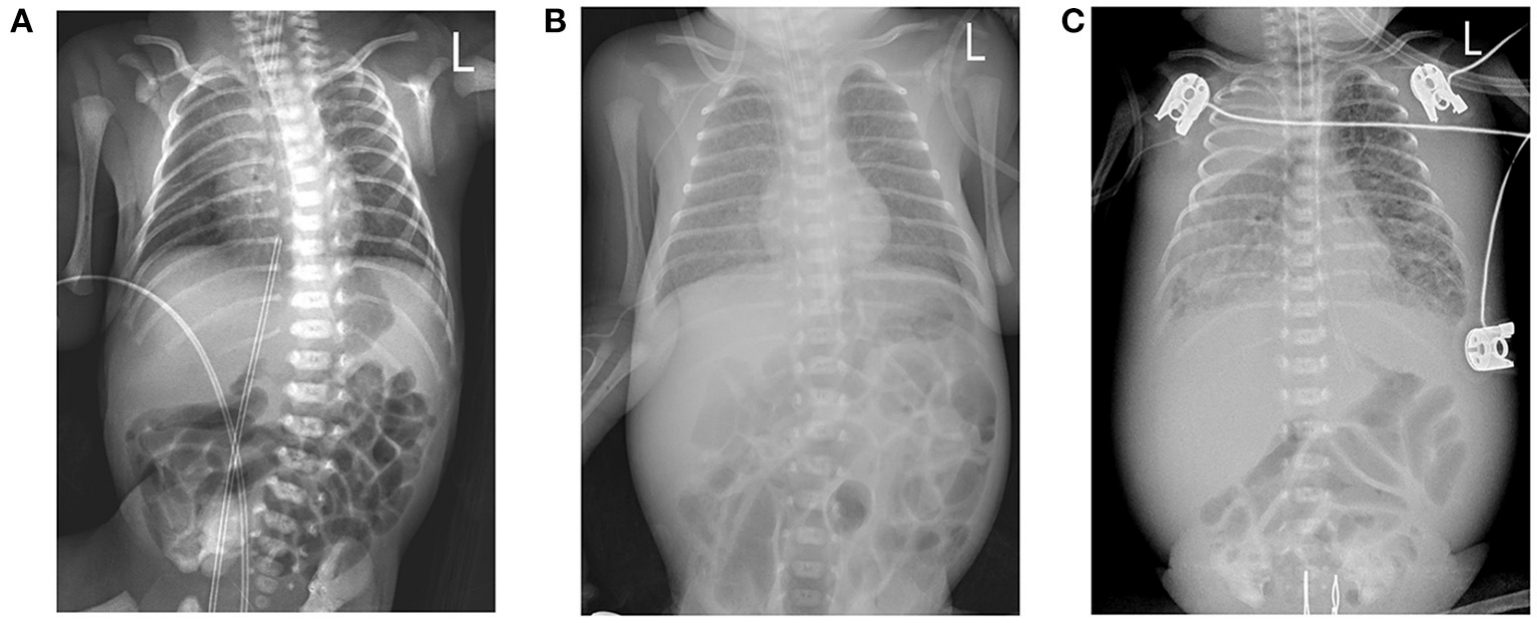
(A) Chest X-ray image shows a bilateral thickening of lung markings, with patchy and blurred shadows in the lower lung fields. (B) Chest X-ray image depicts thickening and blurring of bilateral lung markings, with small patches of fuzzy shadows in the lung fields. (C) Chest X-ray image shows that the texture of both lungs was thickened, increased, and blurred. Large dense shadows of uniform density were observed in the right upper lung field, and small patchy blurred shadows were found in the remaining lung fields. There was a narrow band of increased density in the field of the right lower lung that contained a clear boundary.
